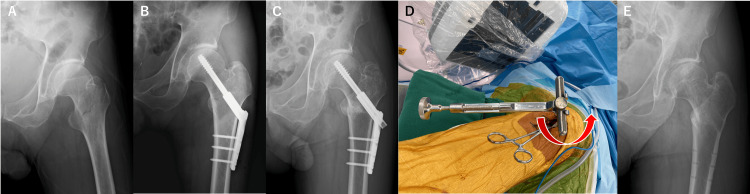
X-ray and intraoperative images of a 58-year-old male patient with an intertrochanteric fracture (AO 31A1.2). (A) Initial radiograph. (B) Radiograph at internal fixation with a keyless-type CHS system. (C) Radiograph at 18 months after internal fixation. (D) By gripping the T-handle with Roman forceps to increase torque, the screw was successfully extracted. (E) Radiograph after implant removal. CHS: compression hip screw
